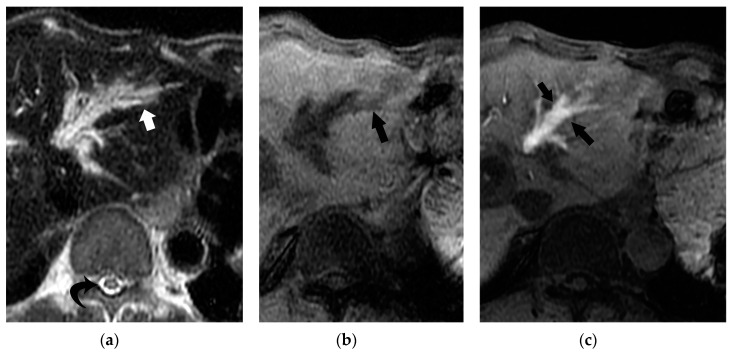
MR findings of BDN. (a) On T2-weighted MRI, BDN (arrow) on the left lateral lobe of the liver shows thick tubular hyperintensity, and the degree of hyperintensity is not so high compared to the cerebrospinal fluid observed in the spinal canal (curved arrow). (b) In the hepatobiliary phase of Gd-EOB-DTPA (EOB), which is 20 min after intravenous (iv) administration, the BDN site shows hypointensity compared to the background liver (arrows). Usually, in this phase, the intrahepatic bile duct shows hyperintensity because of secretion of EOB to the bile. (c) About 70 min after intravenous administration of EOB, the BDN area shows marked hyperintensity (arrow), which represents EOB exuding into the BDN region and being retained at the necrotic area.
